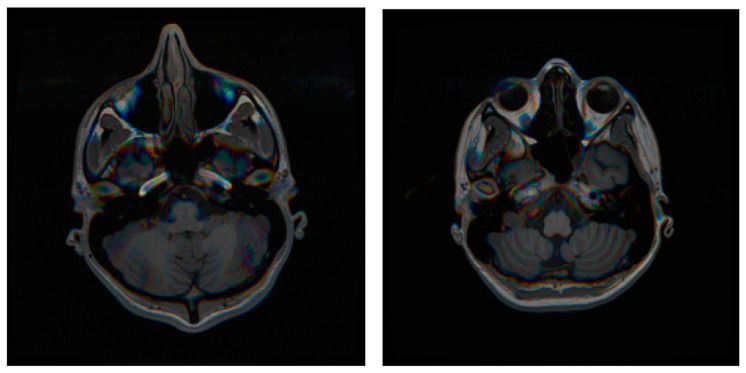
Differences in carotid arteries contrast in brain T1-weighted MR images in two subjects.
